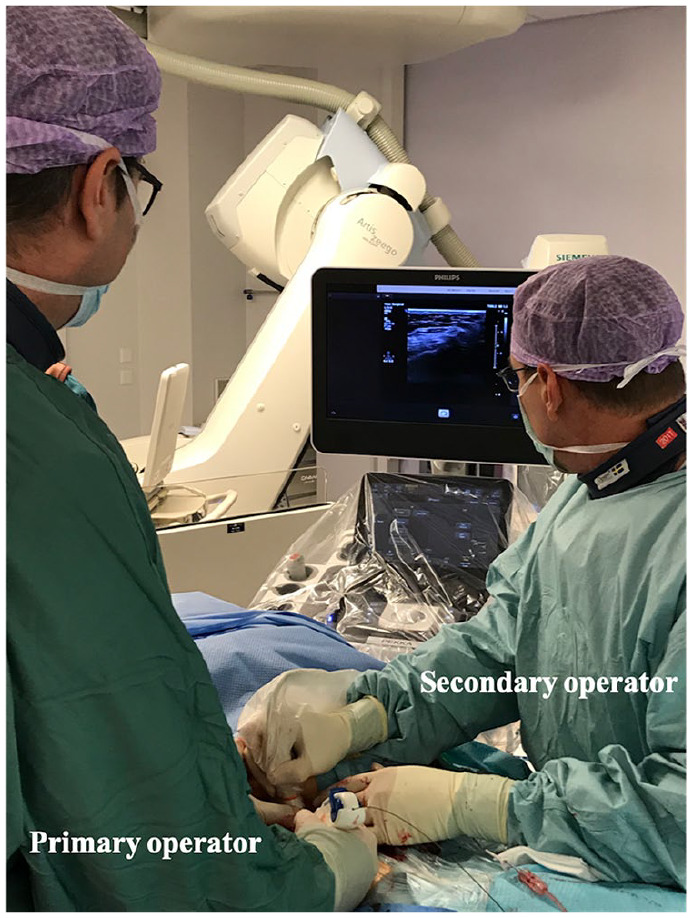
Ultrasound-navigated MANTA method in Helsinki University Hospital. The
